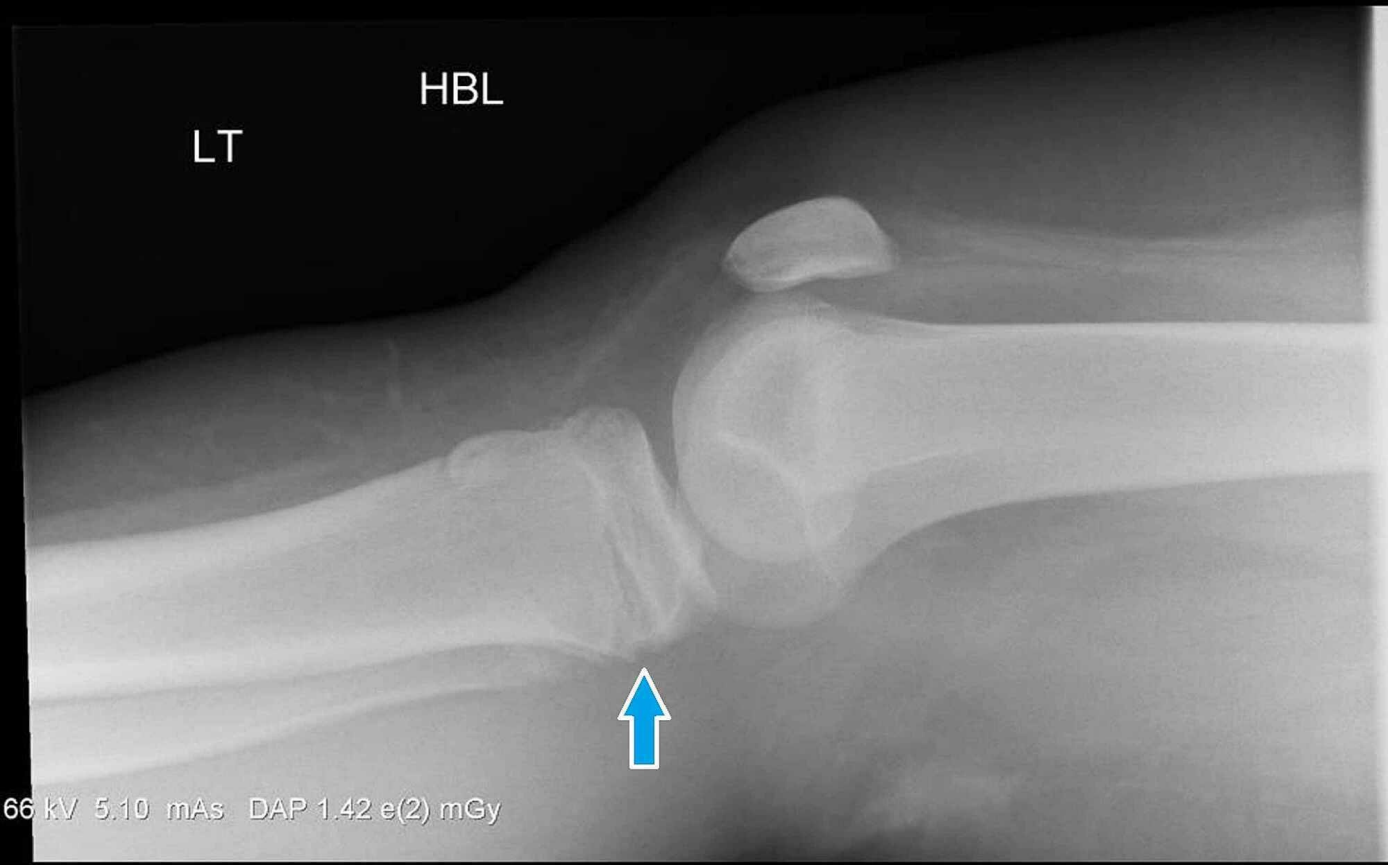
Radiograph of left knee upon initial injury (lateral view)
